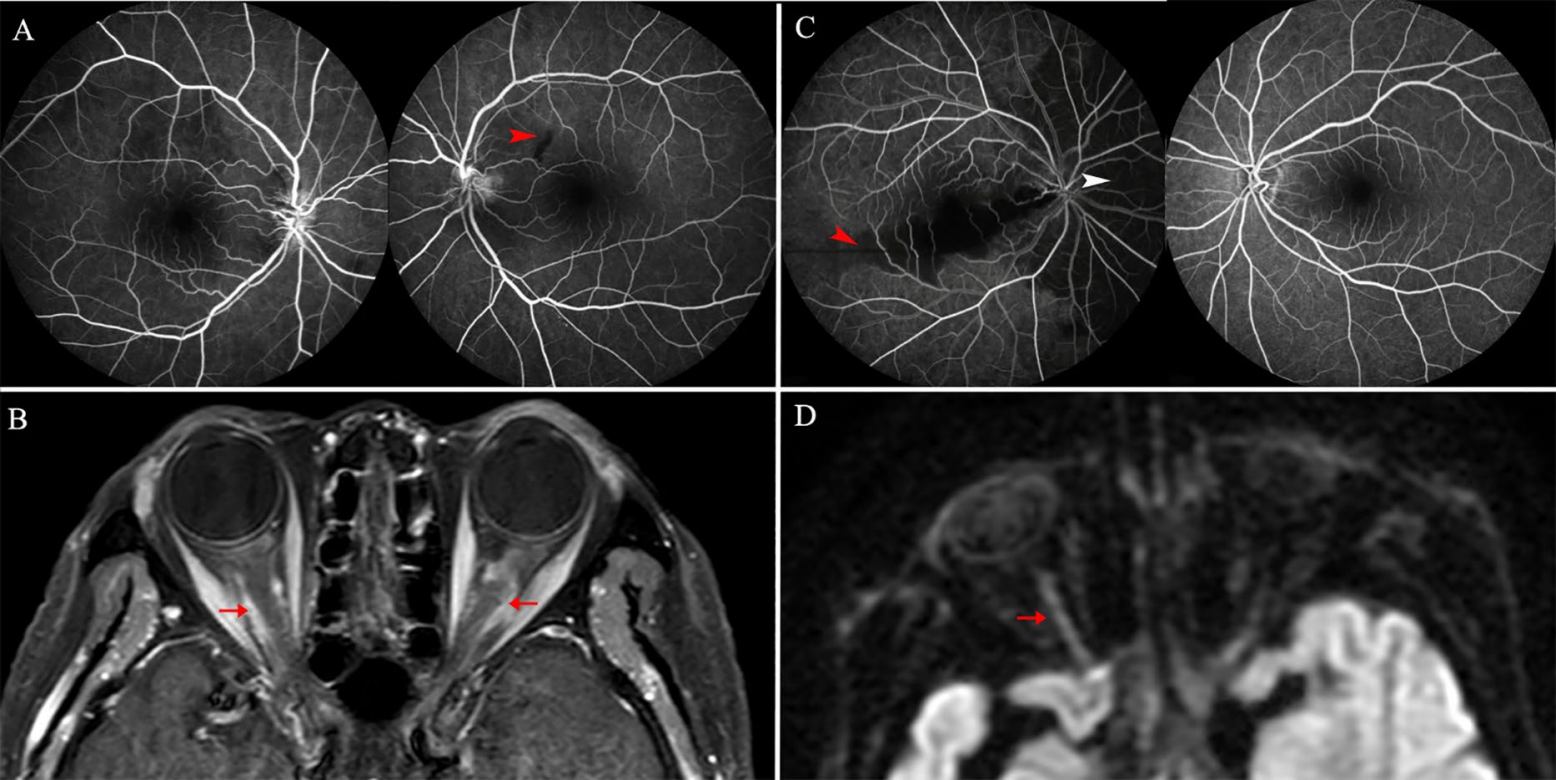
A, B from an 86-year-old woman. A, FA: bilateral disc edema with fluorescein leakage. Lesions in the retinal small arteries result in nerve fiber infarcts. (red arrowhead) B, Axial T1-weight postcontrast MRI shows enlargement and enhancements of the bilateral optic nerve (red arrow). C, D from a 67-year-old man. C, FA: Disc edema, the cilioretinal artery occluded (red arrowhead) and choroidal ischemic area (white arrowhead) presented on the right eye. D, DWI: hyperintense DWI signal (red arrow) was seen in the right optic nerve
